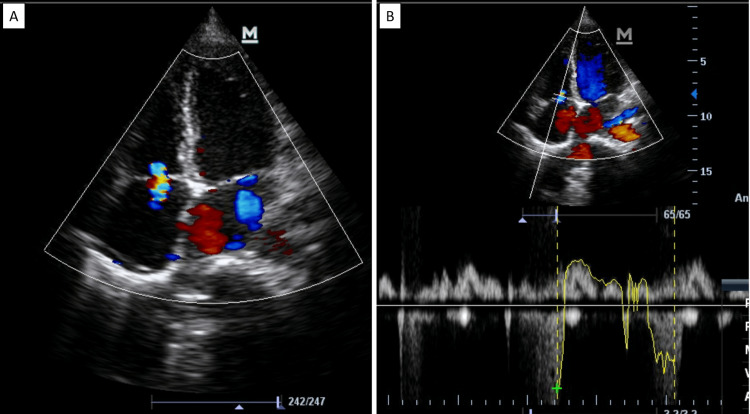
Echocardiogram with apical four chamber view showing dilated left atrium (LA) and left ventricle (LV), global hypokinesia of LV with moderate LV systolic dysfunction
